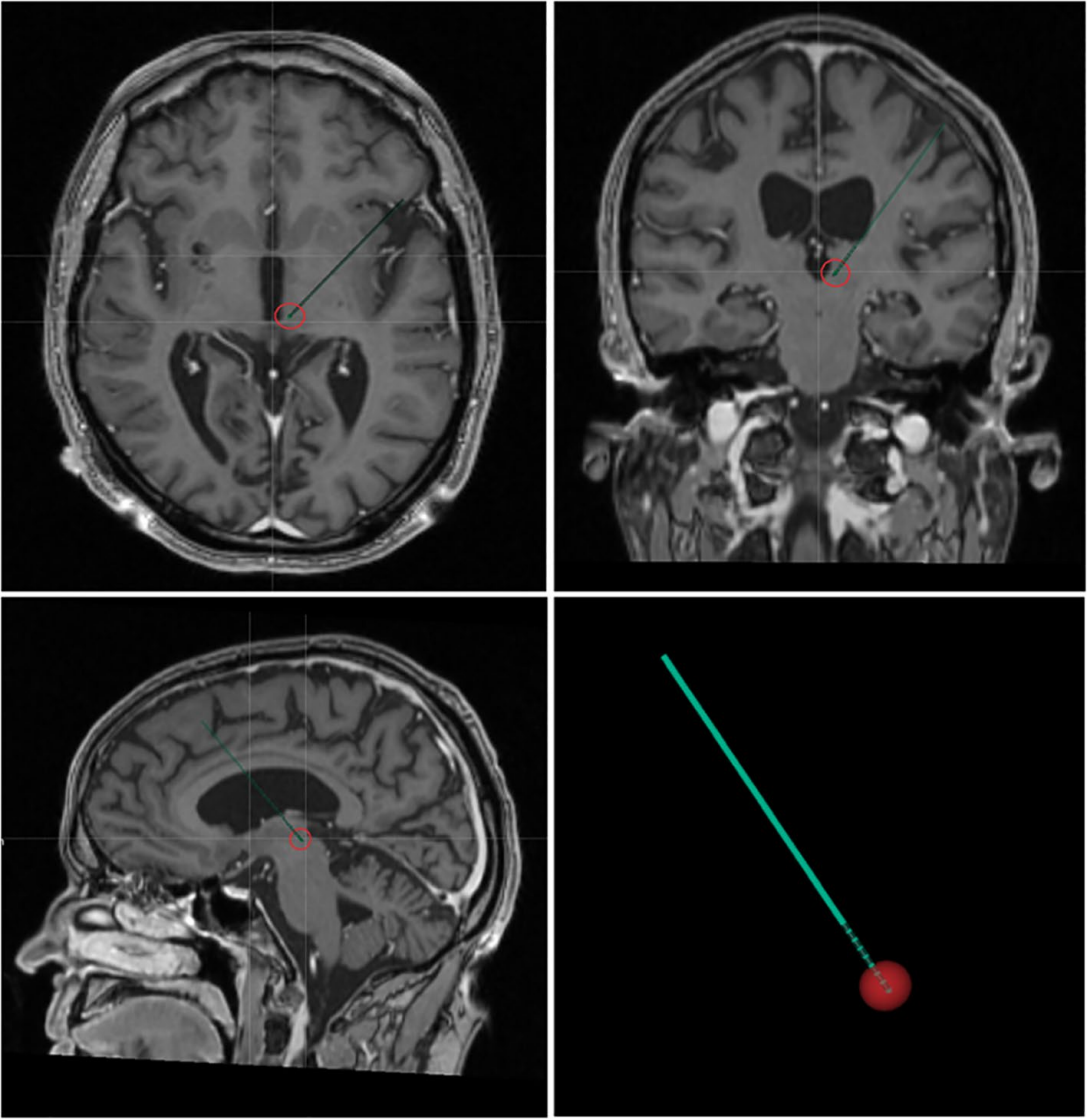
Postoperative lead localization of the right DBS electrode projected in a T1-weighted MRI, showing active contact points in the centromedian-parafascicular complex with its effective electrical field size visualized (bottom right) by calculating the volume of tissue activated in Brainlab’s Guide-XT software module (Brainlab AG, Munich, Germany, version 3.2.0.281). Monopolar settings were used with the lowest contact point as cathode and pulse generator as anode.
